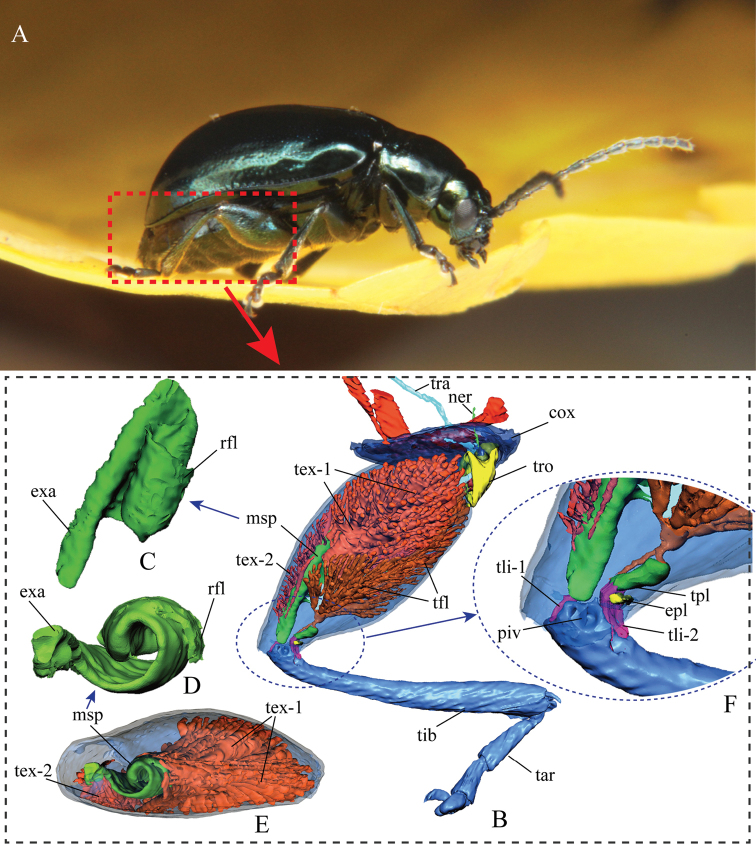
Internal structures of the hind leg of *Alticacirsicola*. A the flea beetle *Altica* sp. on foliage B–F X-ray computer tomography 3D reconstructions of the hind leg of *A.cirsicola*B lateral view of the left hind leg C lateral view of the metafemoral spring D distal view of the metafemoral spring E distal view of the metafemoral spring and tibial extensors F features of the femorotibial joint. Abbreviations: *cox*: coxa; *exa*: extended arm of the metafemoral spring; *epl*: elastic plate; *fem*: femur; *msp*: metafemoral spring; *ner*: nerve; *piv*: tibial pivot of the femorotibial joint; *rfl*: recurve flange of the metafemoral spring; *tar*: tarsi; *tex-1*: primary tibial extensor; *tex-2*: secondary tibial extensor; *tfl*: tibial flexor; *tib*: tibia; *tli-1*: primary tibial ligament; *tli-2*: secondary tibial ligament; *tpl*: Lever’s triangular plate; *tra*: trachea; *tro*: trochanter.
